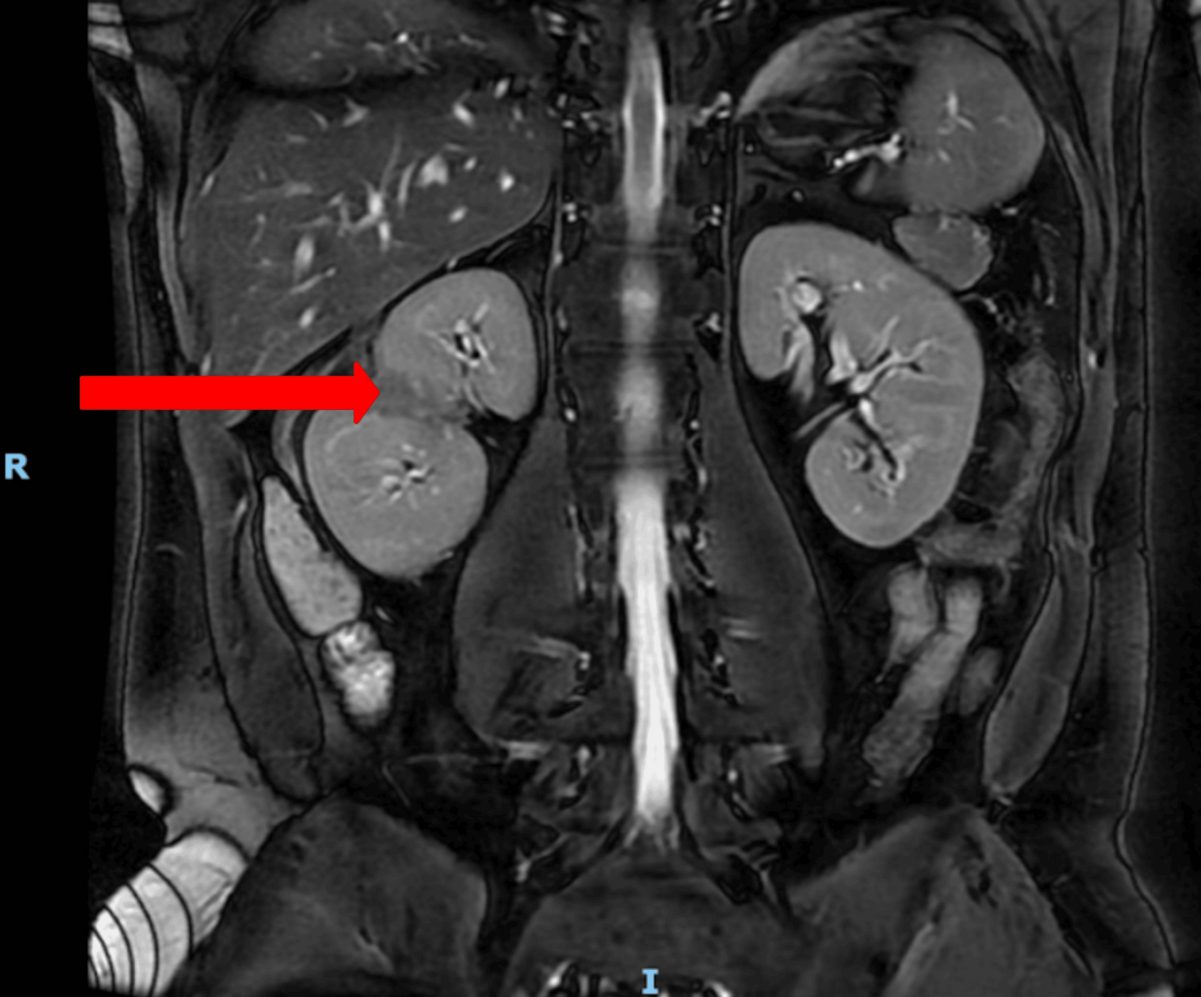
MRI renal (coronal view) taken four months after initial insult The red arrow points to cortical scarring in the interpolar region of the right kidney. The letter R depicts the right-hand-side of the patient. The letter I depicts the inferior aspect of the patient.
